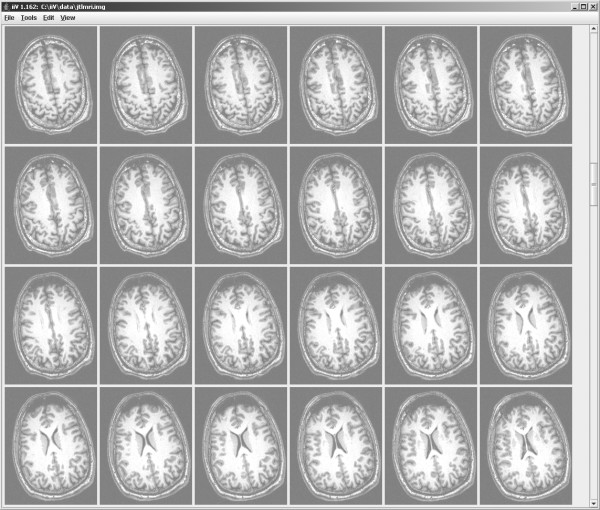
Default display of an MRI stored as signed short. All transverse slices are displayed by default with the full possible short (16-bit) data range mapped to a 256 grey level color map. The maximum value of 32,767 maps to white and the minimum value -32,768 maps to black. Zero maps to the mid grey value which is why the background areas on the slices are grey not black. The MRI actually contained no values less than zero and contrast could be improved by scaling to show positive values only with zero mapped to black as in Fig. 6. By default there are six columns and only 24 of 255 transverse slices fit on the computer display, the rest could be scrolled to for display.
